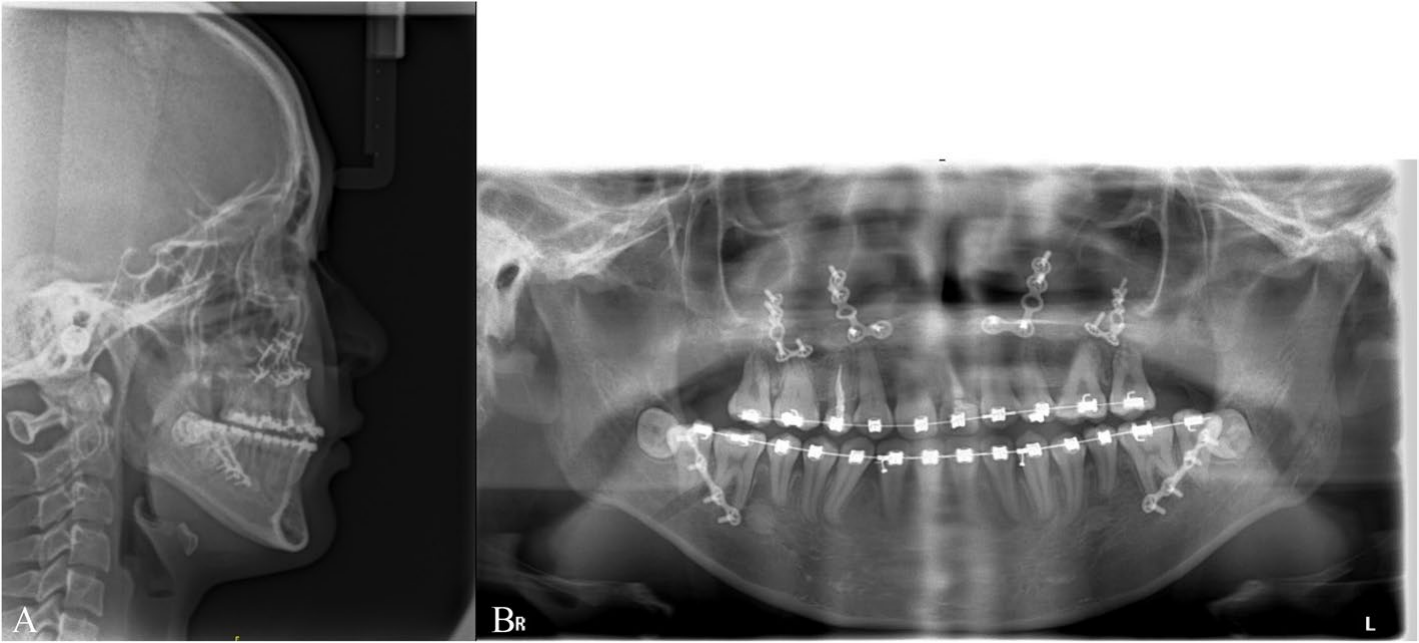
A, B Lateral cephalometric and panoramic radiographs 6 weeks after orthognathic surgery of #3. 22 months after the MASDO procedure, a bimaxillary osteotomy was performed to correct the class III malocclusion and facial asymmetry. The maxilla was advanced by 1 mm and the left side moved downward 1.5 mm to correct the canting of the occlusal plane. The amount of mandibular setback was approximately 4.0 mm on the right side and 2.5 mm on the left side
